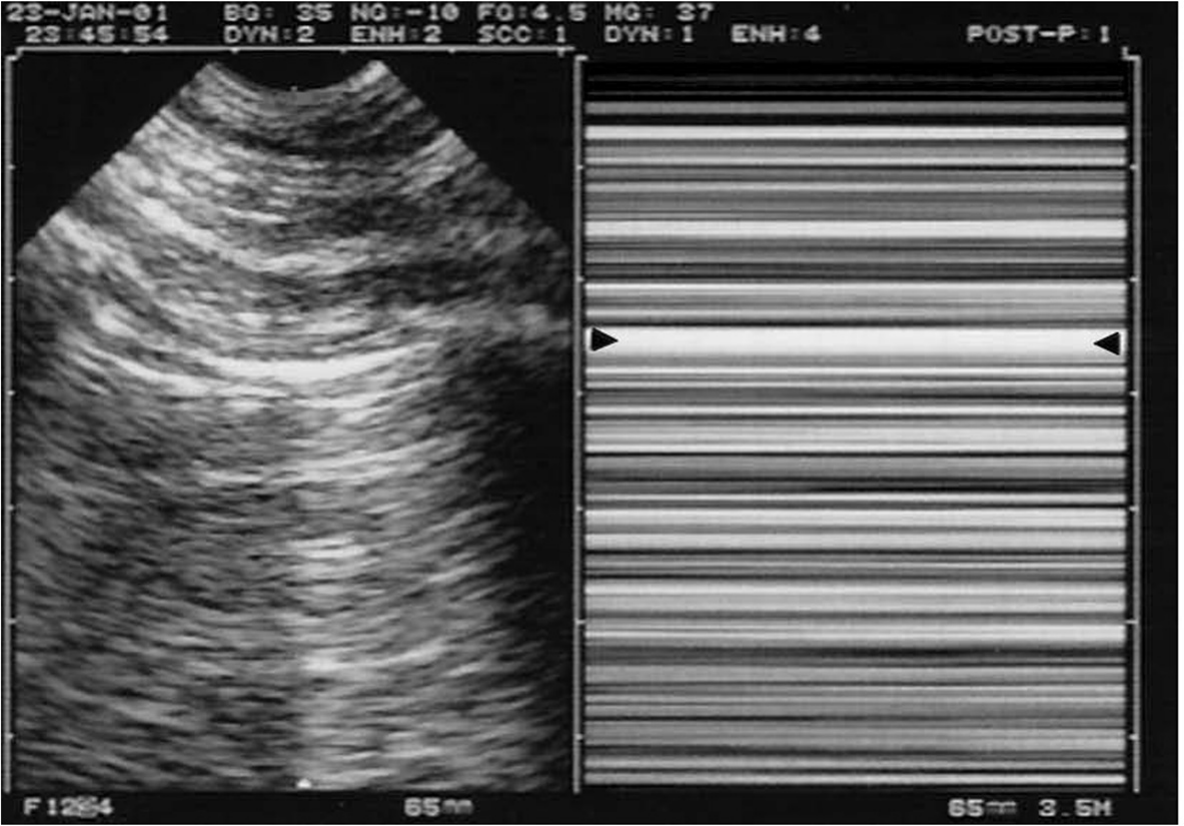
Pneumothorax and the stratosphere sign. Left: same pattern as in Figure 2, i.e., pleural line with A-lines, indicating gas below the pleural line. Not visible on the left image, lung sliding is totally absent. Right: here on M-mode, the abolition of lung sliding is visible through the stratosphere sign (which replaces the seashore sign) and indicates total absence of motion. This suggests pneumothorax as a possible cause (see others in text). Arrows: location of the pleural line. The combination of abolished lung sliding with A-lines, at the anterior chest wall, is the A’-profile of the BLUE-protocol (as opposed to the A-profile, where lung sliding is present, ruling out pneumothorax). Extract from “Whole body ultrasonography in the critically ill” (2010 Ed, Chapter 18), with kind permission of Springer Science.
